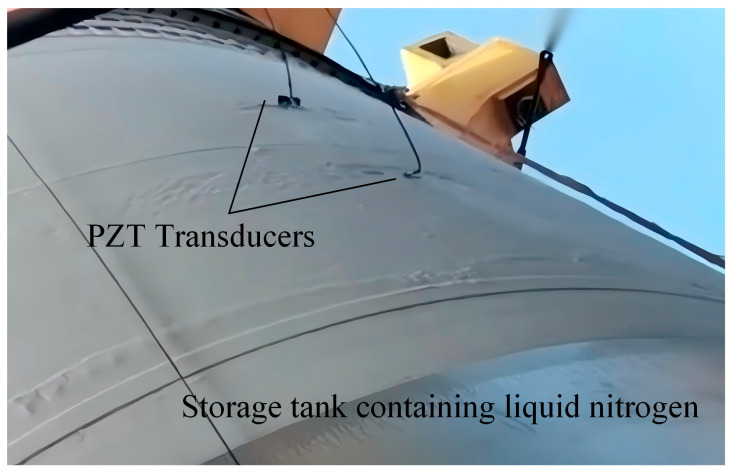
On-site experimental setup for ultrasonic guided wave monitoring of the aerospace storage tank under cryogenic conditions.
